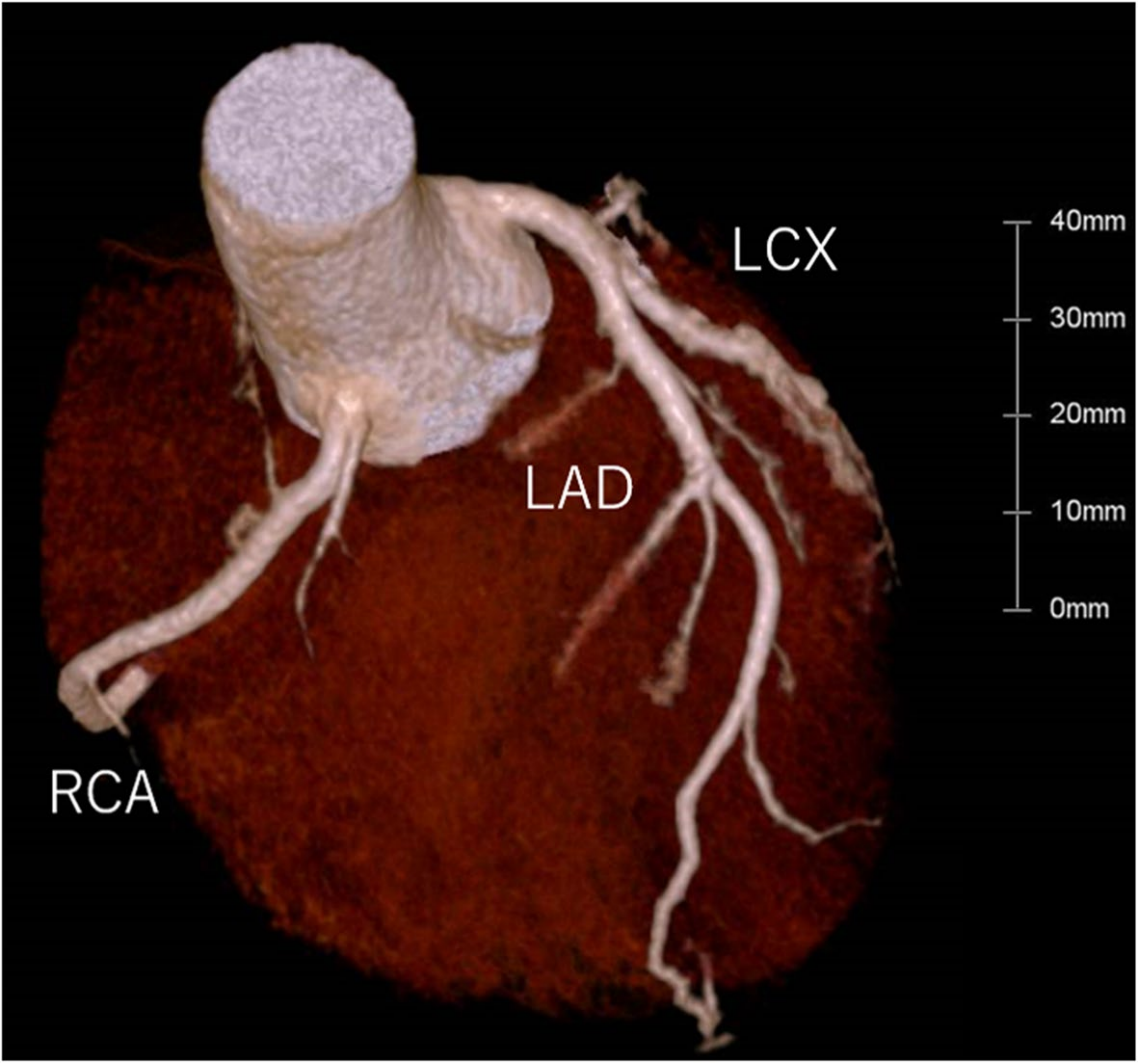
Coronary artery computed tomography. RCA, right coronary artery; LAD, left anterior descending artery; LCX, left circumflex coronary artery. There were no significant stenotic lesions observed in the coronary arteries
